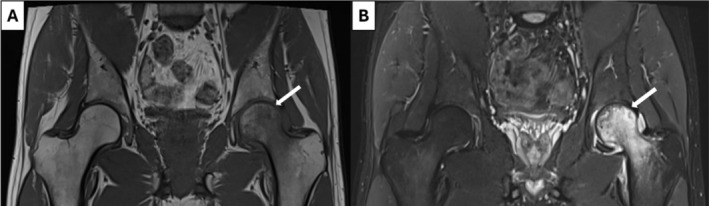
Magnetic resonance imaging (MRI) of the bilateral femoral heads. (A) T1‐weighted image demonstrating a limited subchondral linear‐shaped low signal intensity lesion in the left femoral head. (B) T2‐weighted fat‐saturated image showing corresponding heterogeneous signal changes and a possible “double‐line sign,” consistent with avascular necrosis.
